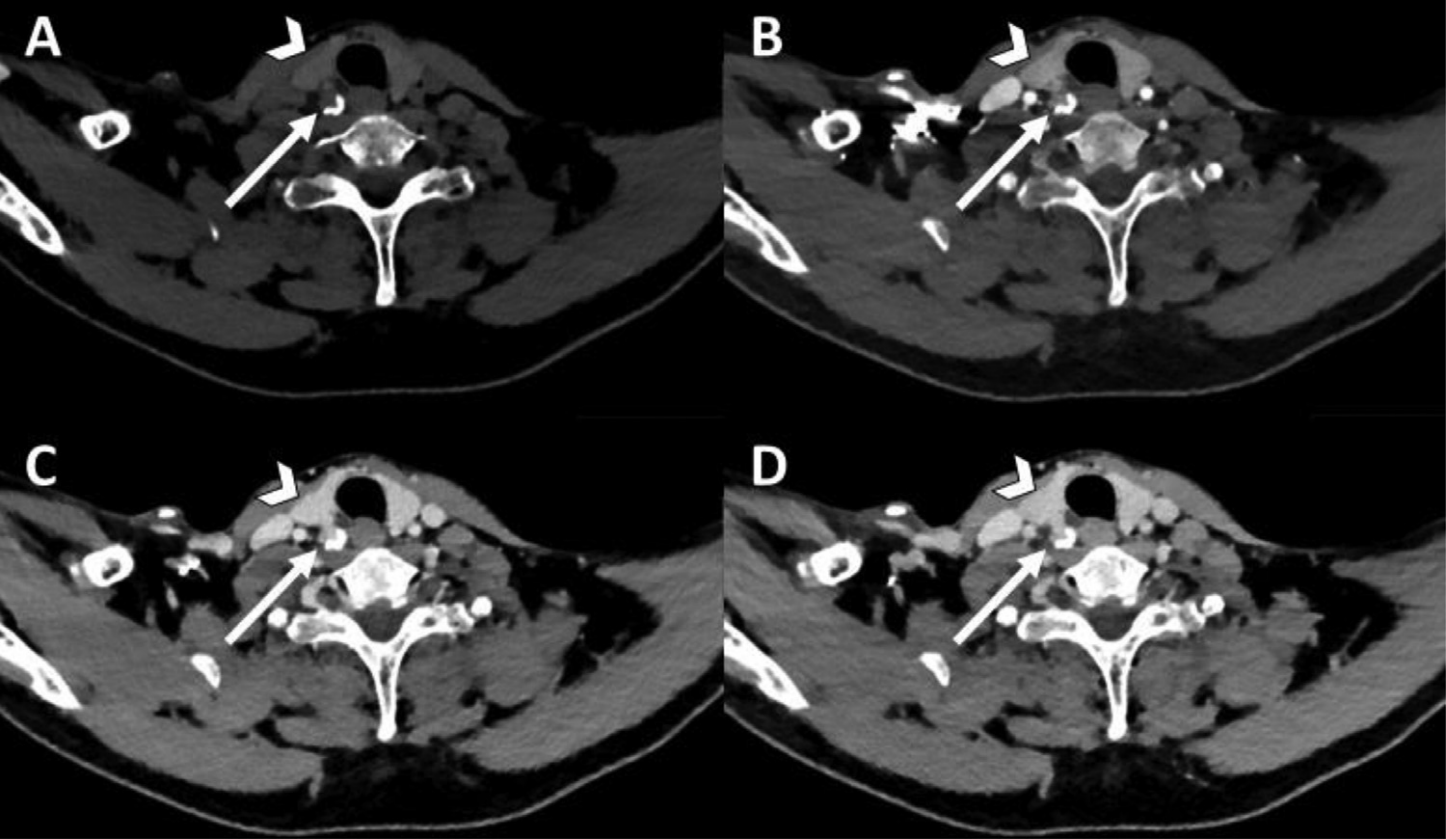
Transversal 4-dimensional CT with nonenhanced (A), arterial (B), venous (C) and delayed venous (D) phase of patient 1. The arrowhead is the thyroid gland. The arrow shows the partially calcified and cystic degenerated parathyroid adenoma, which was mistaken for a thyroid nodule. On the nonenhanced phase, it subtly shows that the noncalcified part shows lower attenuation than the thyroid gland, with arterial enhancement on the arterial phase. This was not reported at first, but after the subtraction map, the arterial enhancement was more clearly visible.
